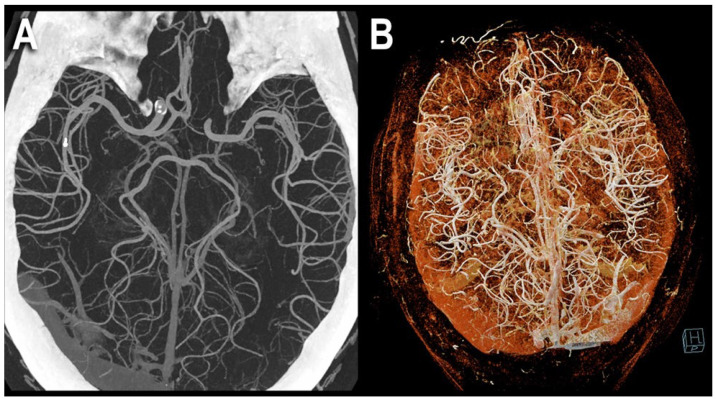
PCCT angiography of the head: 3D cinematic volume rendering display. The figure shows a PCCT angiography of the brain with ultra-high resolution of 100 microns (source dataset; matrix 1024 × 1024; slice thickness/increment 0.2/0.1 mm; convolution kernel B60f; radiation dose comparable to equivalent CT angiography of the head with comparable Dual-source CT of the 3rd generation) using volumetric 3D Maximum Intensity Projection (MIP) (A) and cinematic volume rendering (B). To be noted is the massive increase in the density of the small arterial vessels displayed.
